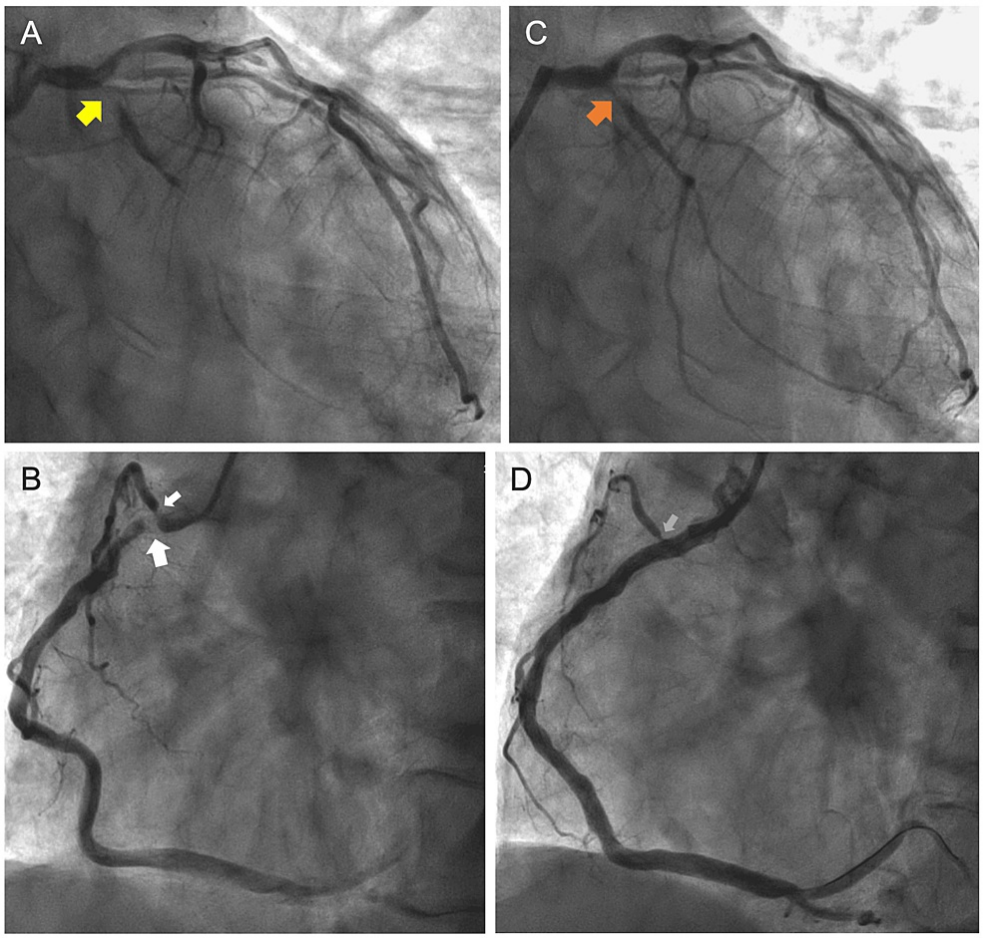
Coronary angiography Coronary angiograms reveal severe narrowing without thrombi in the proximal left circumflex coronary artery (LCCA) (yellow arrow) and proximal right coronary artery (RCA) (large white arrow), including the right ventricular branch (small white arrow); however, only moderate lesions with no significant stenosis are found in the left anterior descending coronary artery (A and B). An intravascular ultrasonography-guided 3.0 × 20 mm everolimus-eluting platinum–chromium coronary stent is successfully deployed to the proximal RCA and post-dilated using a 3.0-mm noncompliant balloon without residual stenosis (D), although the ostium of the right ventricular branch remains stenotic (gray arrow). In contrast, a 2.5 × 20-mm drug-coated balloon is dilated at the proximal LCCA after pre-dilation using a 2.5-mm noncompliant balloon, resulting in residual stenosis (orange arrow) (C). The images in panels A and B represent the anteroposterior and 30° caudal views, and those in panels C and D represent the 50° left anterior oblique view.
